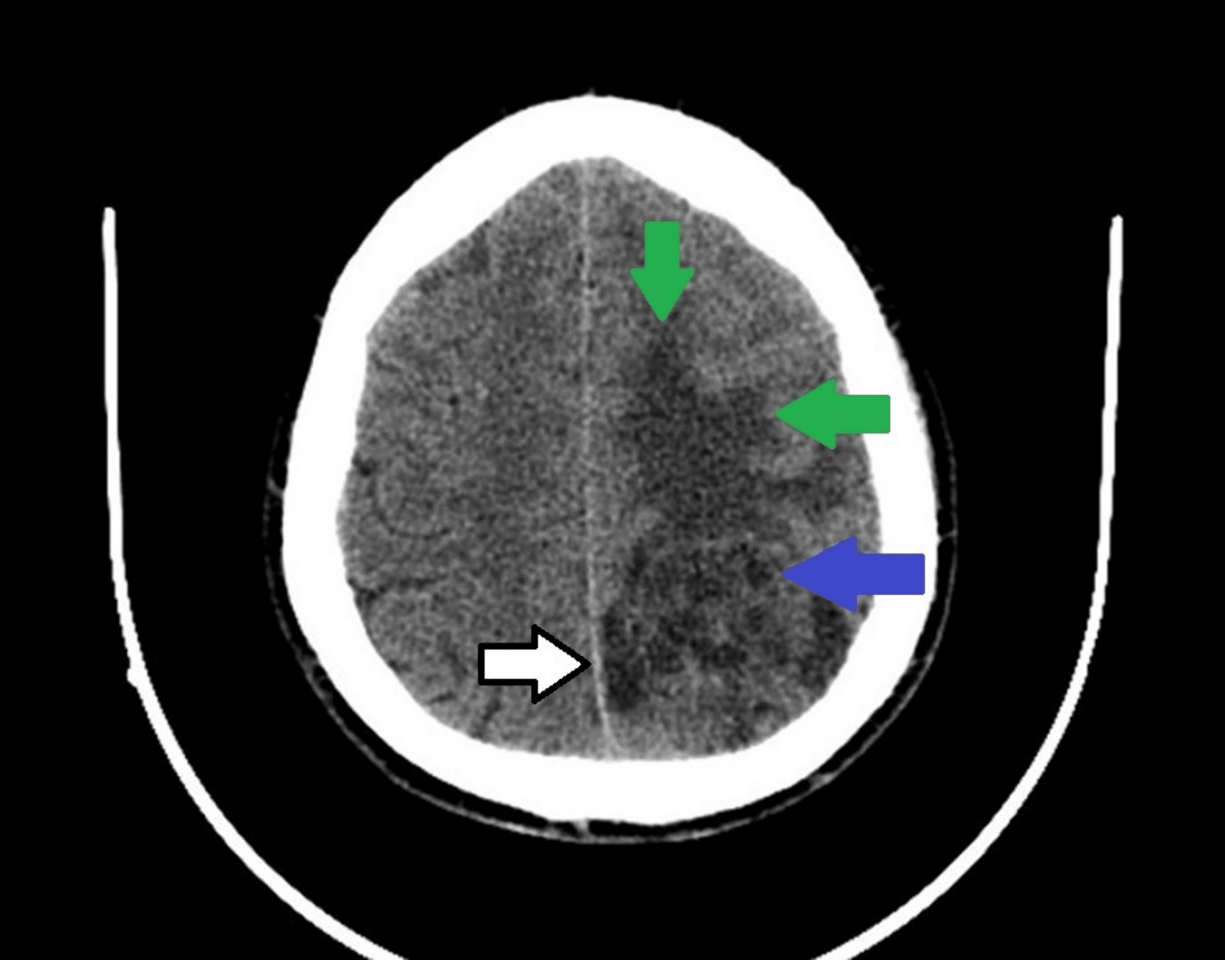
Non-contrast head CT scan showing a left parietal mass (blue arrow) with marked perilesional edema (green arrows) and mild rightward midline shift (white arrow with a black outline).
